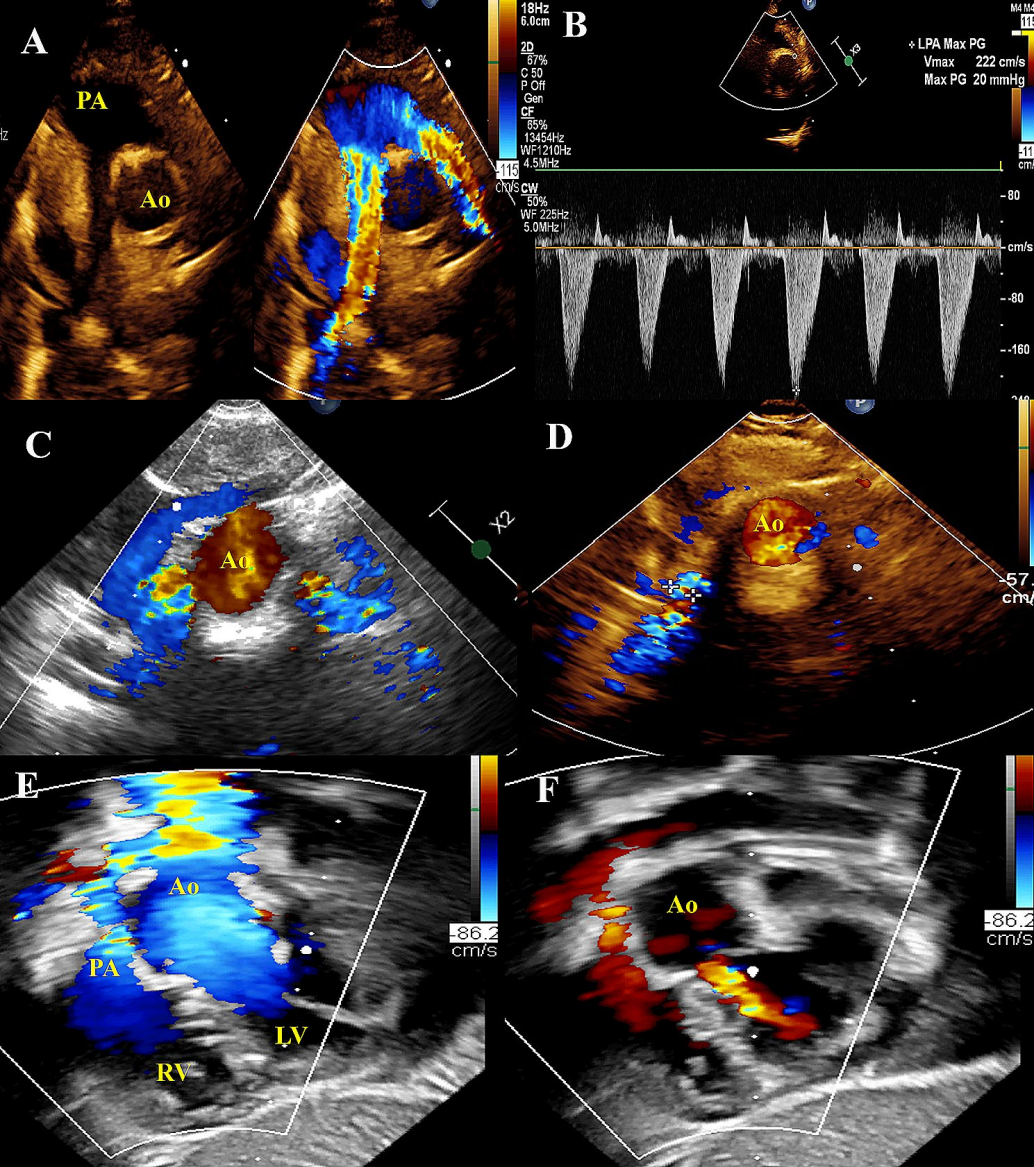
Echocardiographic images of some of the study cases. (A) Both LPA and RPA branches are clearly seen draping around the neo-aorta in suprasternal view in the first patient. (B) Continuous wave Doppler showing pressure gradient of 20mmhg across LPA in the same first patient. (C) Distal parts of both PAs are seen with no visualization of the bifurcation and proximal parts in suprasternal view in the second patient. (D) Third case with only the lower part of RPA is seen as small turbulent branch with LPA is non-visualized in the suprasternal view, although both are described as normal in MSCT. (E, F) Subcostal view from a fourth patient showing a dilated aortic root compressing the main PA anteriorly with aortic and pulmonary regurgitation is seen in diastole. Ao: neo-aorta, PA: neo-main pulmonary artery, RV: right ventricle, LV: left ventricle
